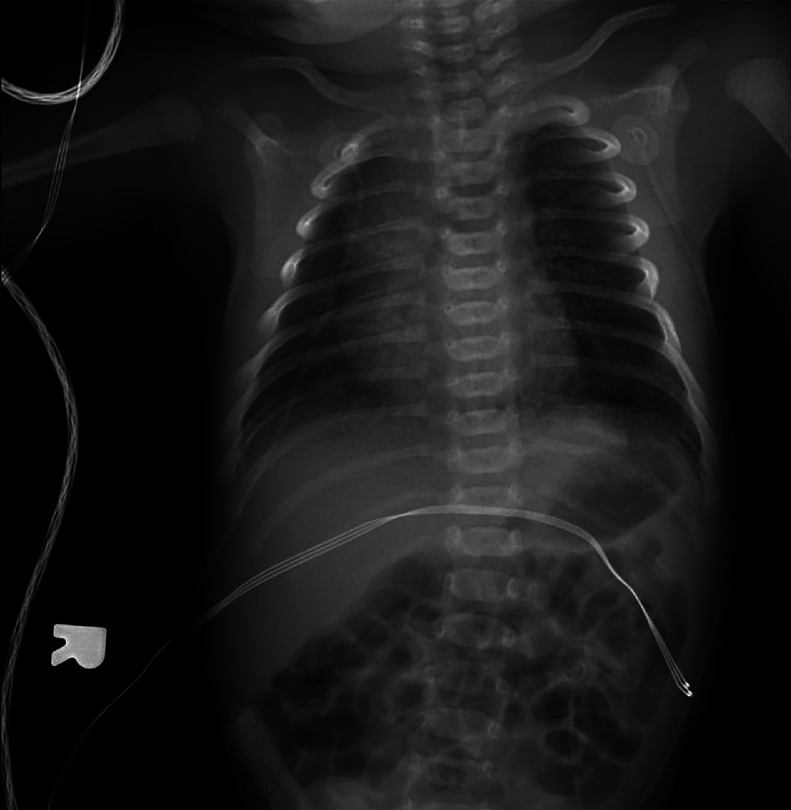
Frontal radiograph of the chest and abdomen on Day 1 of life demonstrating no evidence of cystic lung lesions or dextroposition of the fetal heart.
